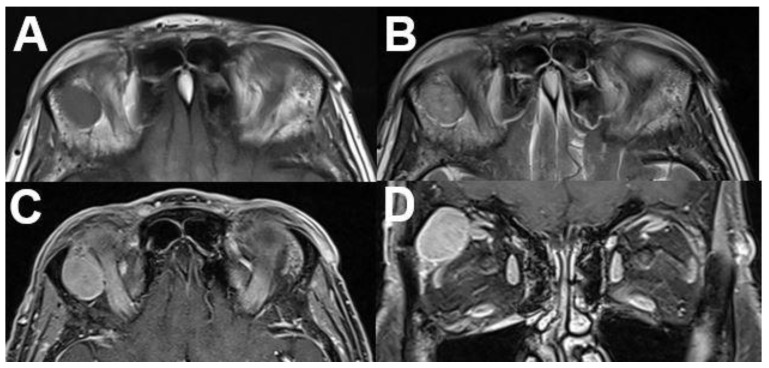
Neurofibroma.* MRI of a 59-year-old female patient with a tumor of the right-sided upper orbit. The tumor is located between the superior and lateral rectus muscle, showing T1-isointense (A) and slight T2-hyperintense (B) signal compared to the muscles. It shows heterogeneous contrast enhancement on axial (C) and coronal (D) views. In this case, diagnosis was proven after resection.
